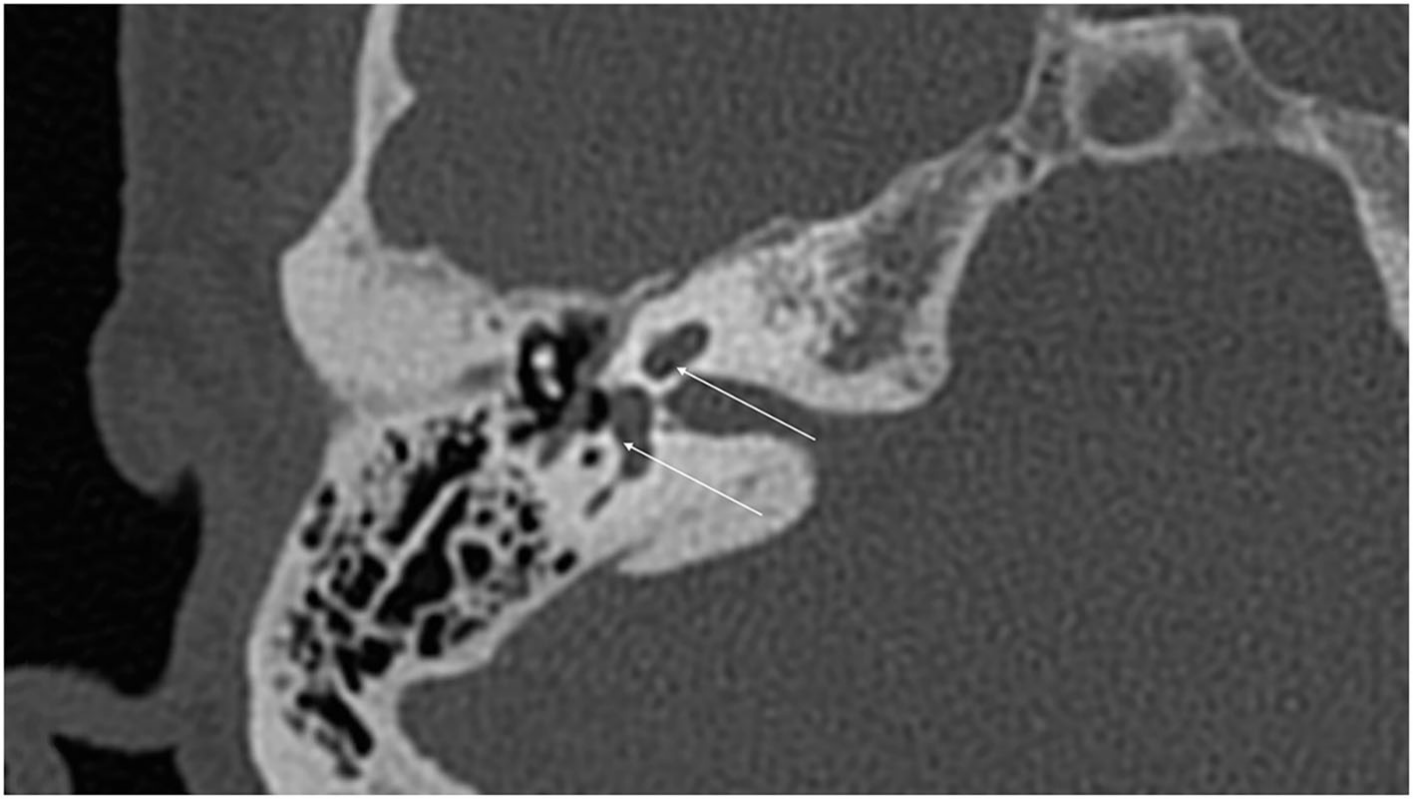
False positive hypodensities (arrows) seen in the cochlea on routine CT of temporal bone.
